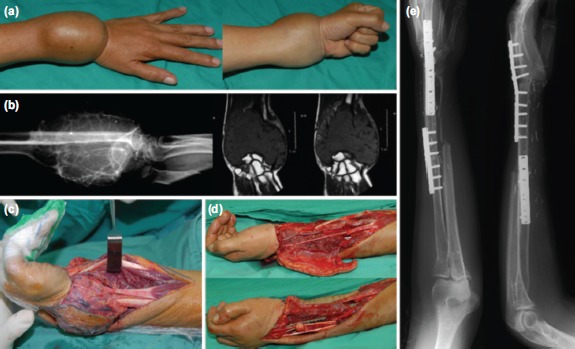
Wrist fusion in a 42 year-old man with long standing swelling for five years. (a) Pre-operative photo. (b) Pre-operative radiograph and MRI revealed expansile septated lesion involving the entire distal radius with distal extension to proximal row of carpus and distal ulna. (c) Resection of radius and ulna with proximal row carpus. (d) Intra-operative photo showing the defect post-resection (top) and reconstruction with vascularised fibular graft (bottom). (e) Plain radiograph taken eight years post-operatively with stable wrist fusion with good hand grip.
